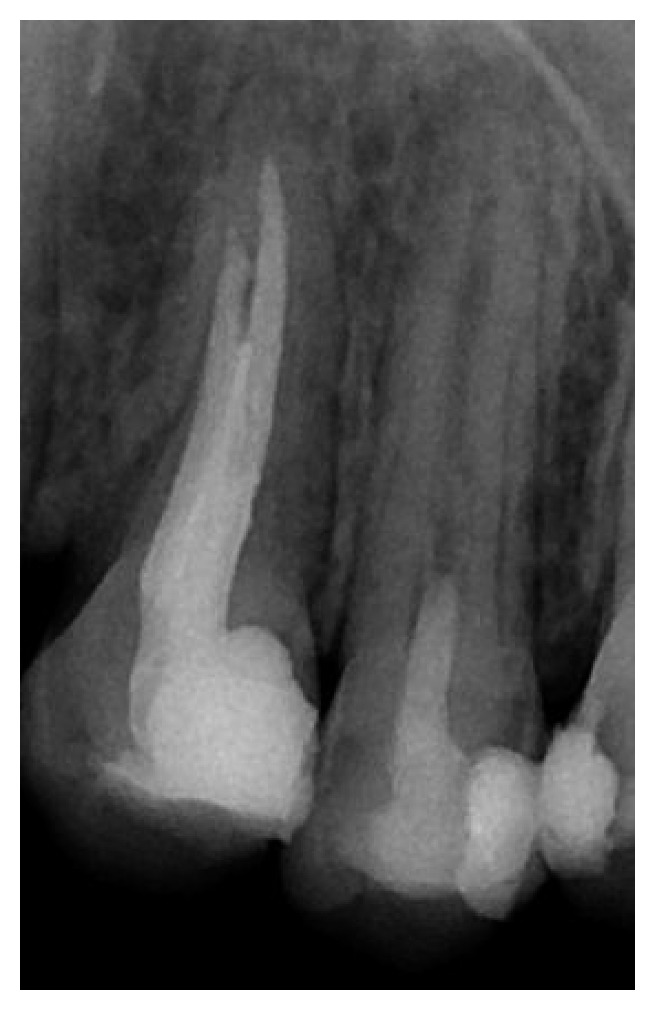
Thirty-month follow-up radiograph showing a normal periapical image.
